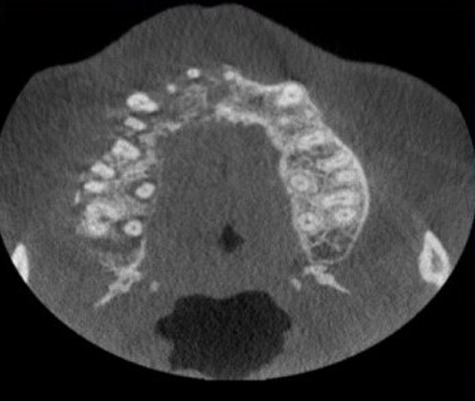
axial cone beam computed tomography (CBCT) view shows loss of buccal cortical bone
